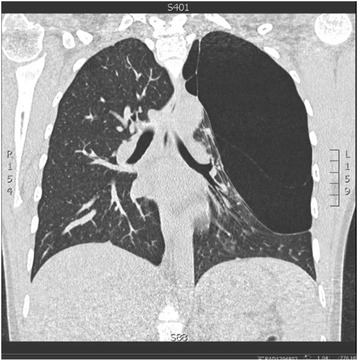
Pre-operative high resolution CT scan, showing the massive bulla (17 × 12 cm) of the *left* upper lobe with compression of the remaining lung parenchyma
